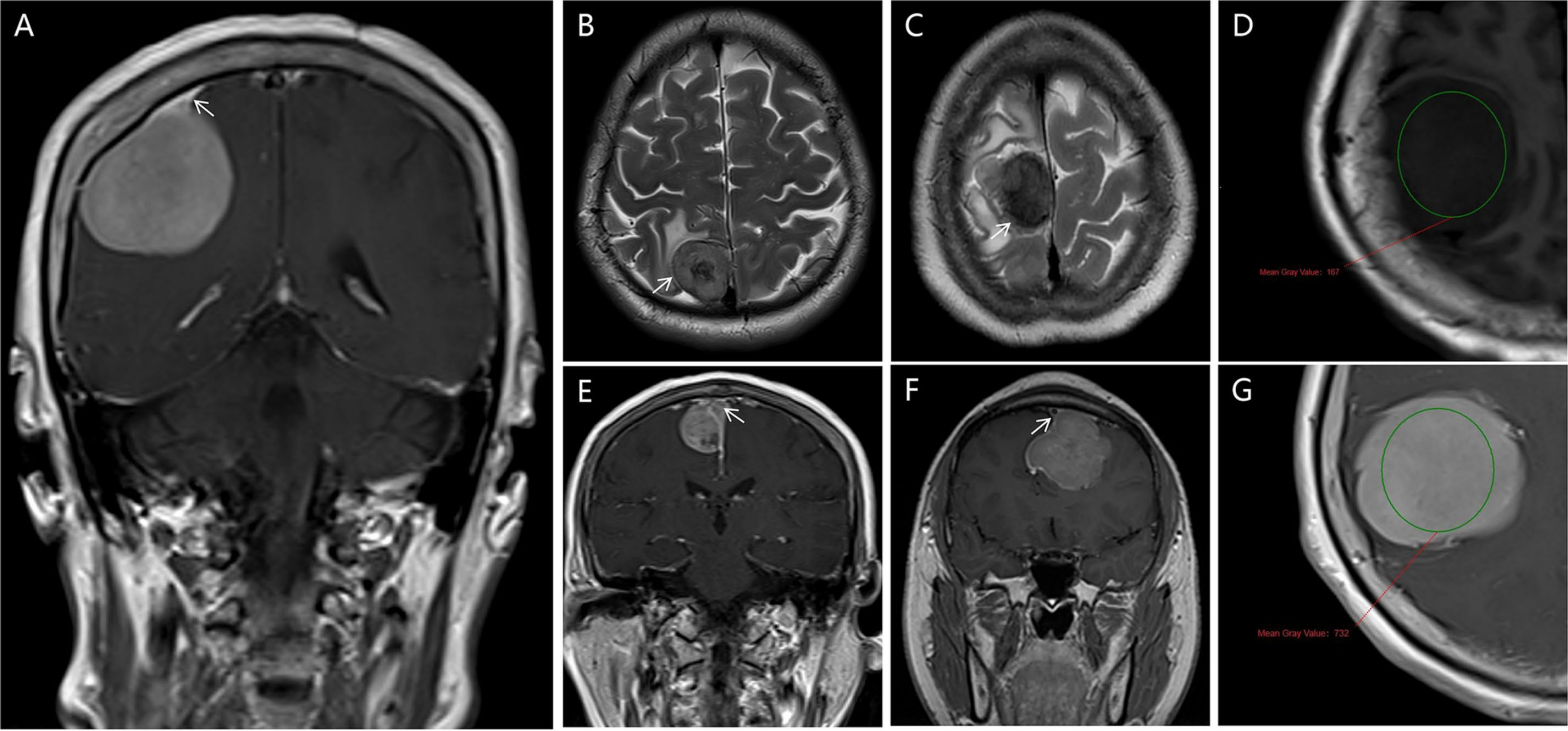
Radiological features are depicted in this study. (A) Dural Tail Sign in Weighted T1 MRI (white arrows pointed); (B,C) shows a CSF cleft in T1, and T2MRI image (white arrows pointed); (D,G) the degree of tumor enhancement was assessed by measuring the difference in grayscale values on T1WI before and after contrast enhancement of the solid tumor components (circles place); (E,F) the extent of venous sinus invasion was evaluated by analyzing the relationship between the tumor and the venous sinuses on MRV images (white arrows pointed).
